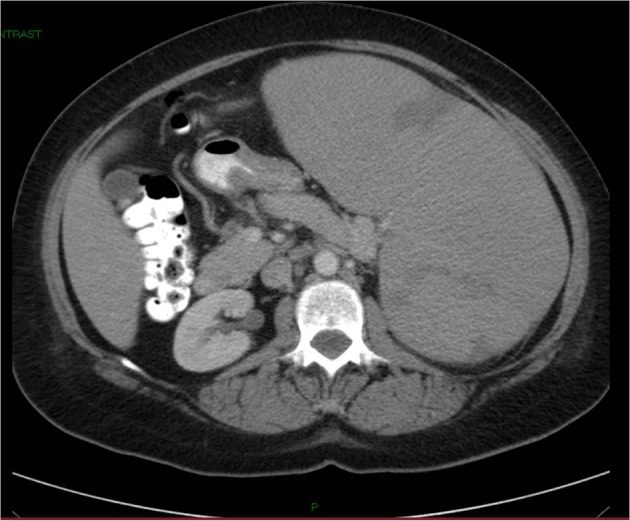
CT of abdomen and pelvis without contrast showing massive splenomegaly measuring 22.8 cm.
